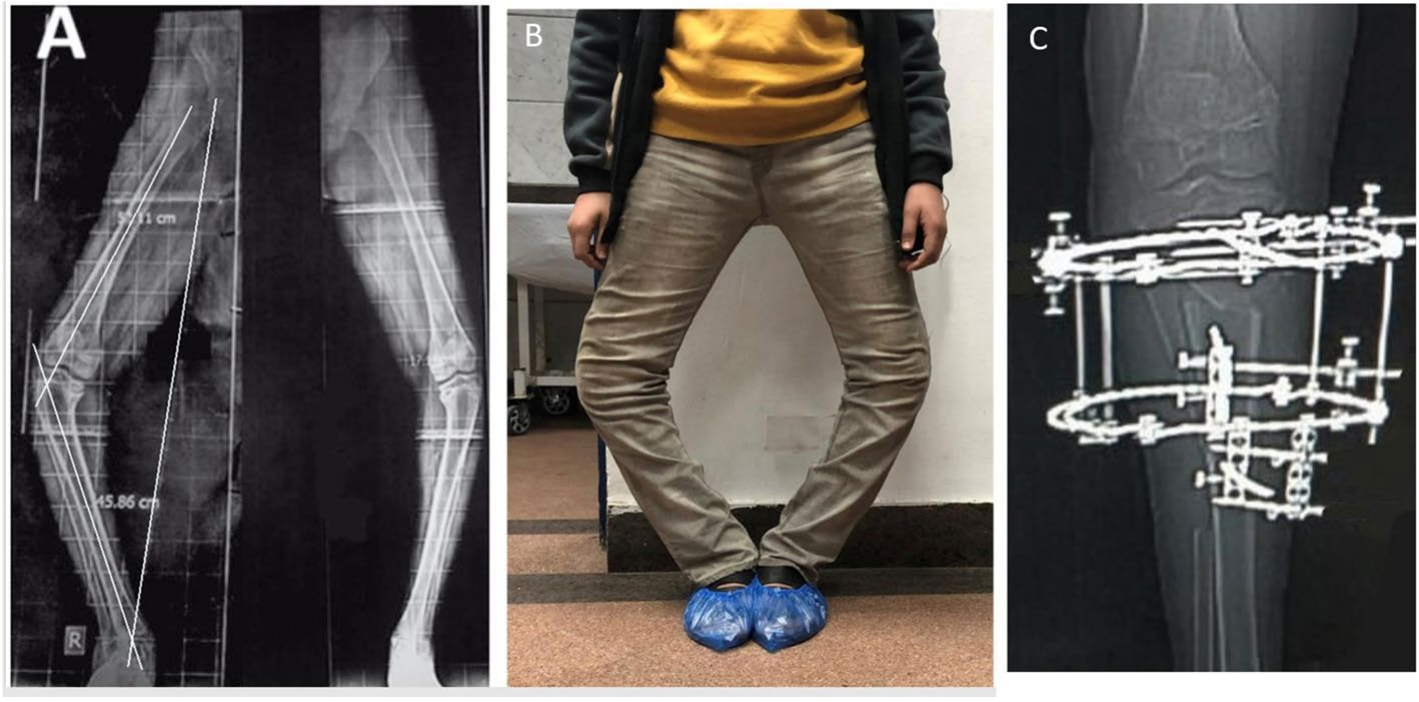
Preoperative full leg length scanogram (TFA -48°) (A) and photograph (B) of a 15-year-old male with bilateral severe late-onset tibia vara and ankle valgus. C Post-operative radiograph after knee deformity correction showing the osteotomy
